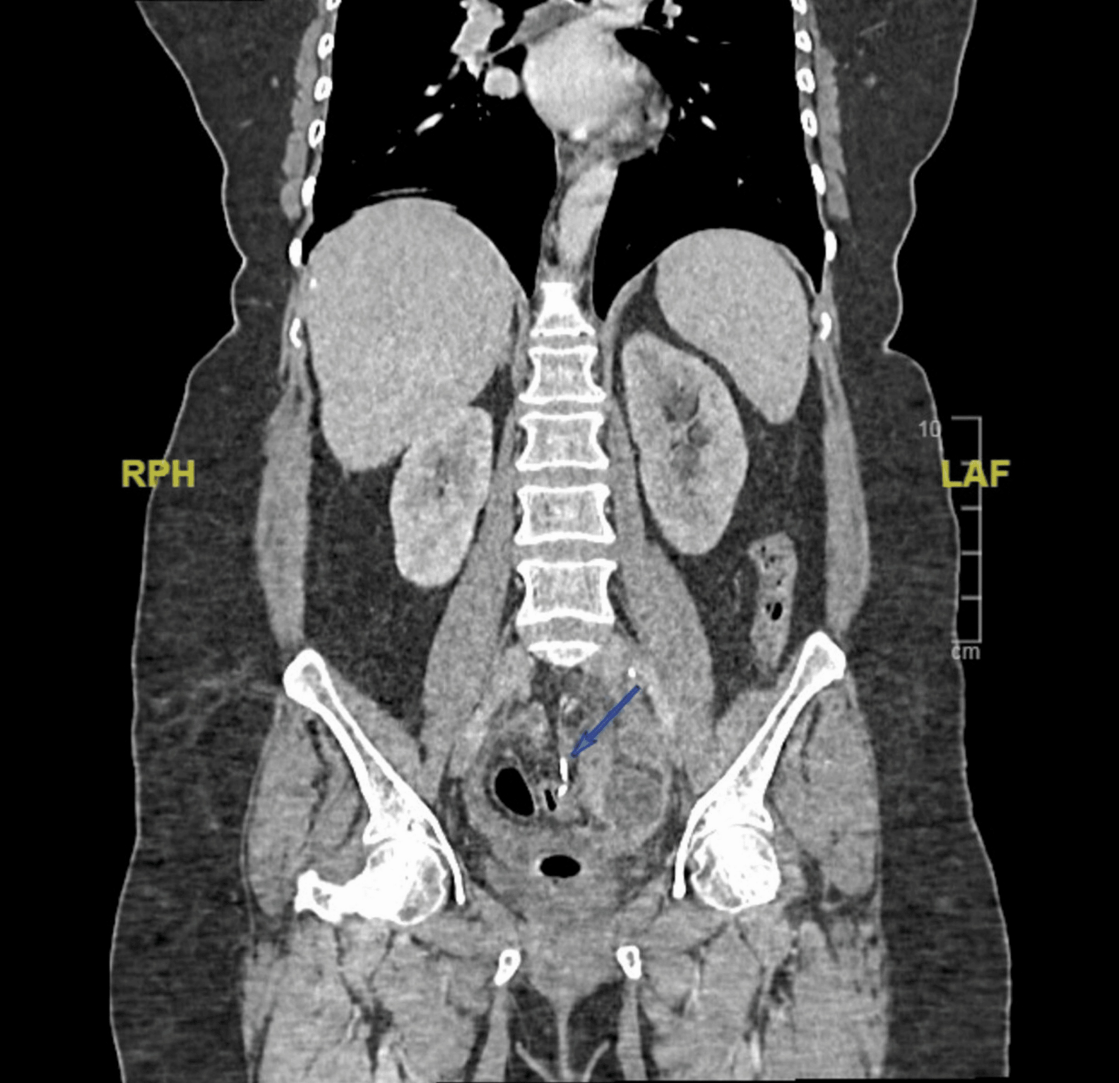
Coronal section of CT imaging showing the hyperdense foreign body.
